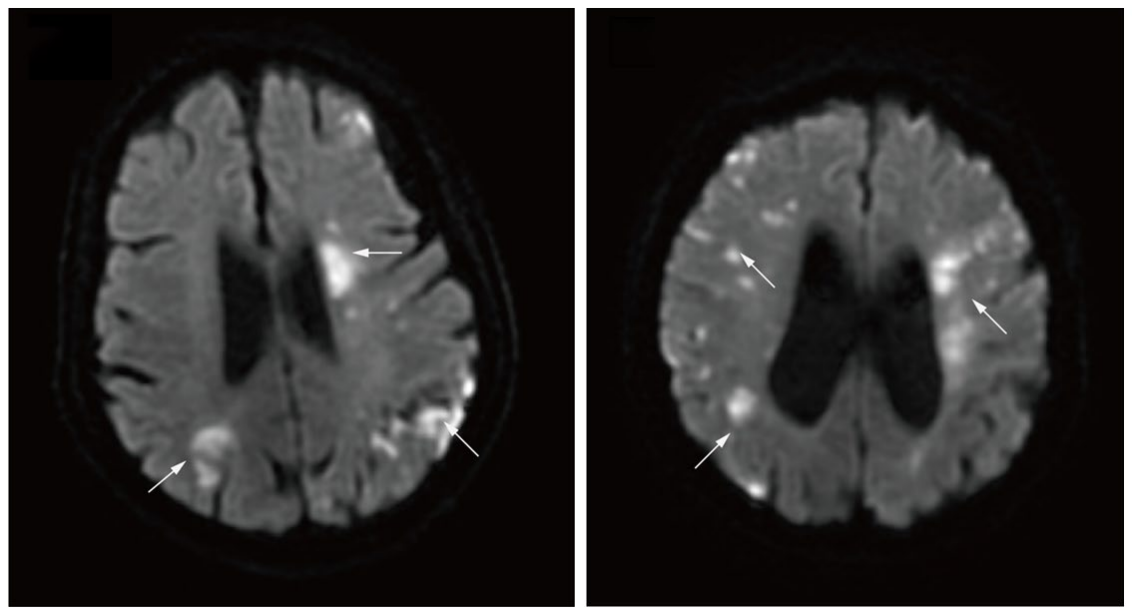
Diffusion-weighted magnetic resonance imaging demonstrating multiple high intensity areas (arrows) in all three major arterial territories of the brain.
